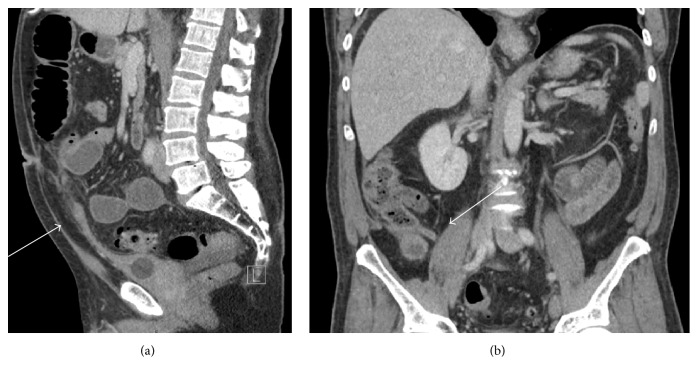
CT scan demonstrating a Foley catheter in the decompressed bladder with (a) demonstration of a urachal cyst connection from the anterior reflection of the bladder to the umbilicus with dilated small bowel loops and adjacent fat stranding and (b) a normal appearing posterior appendix off the cecum.
